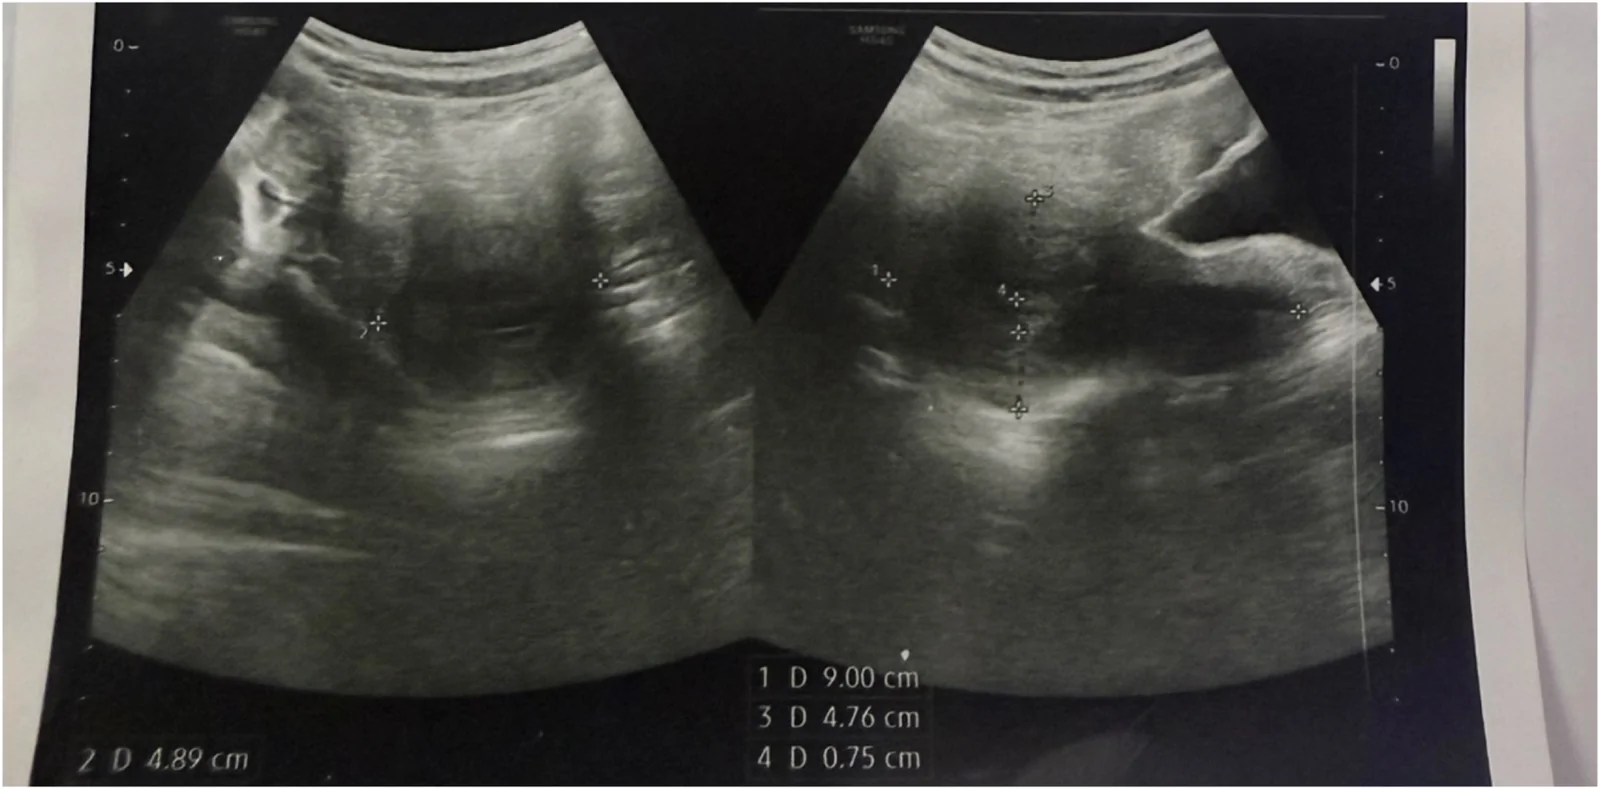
Ultrasonography (USG) of the abdomen and pelvis showing no abnormal findings.
